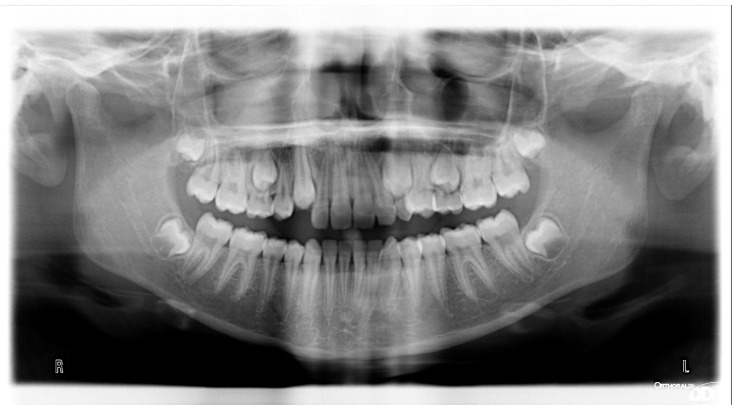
Radiographs of the subject at the screening. Orthopantomography X-ray before treatment (10-year-old).
